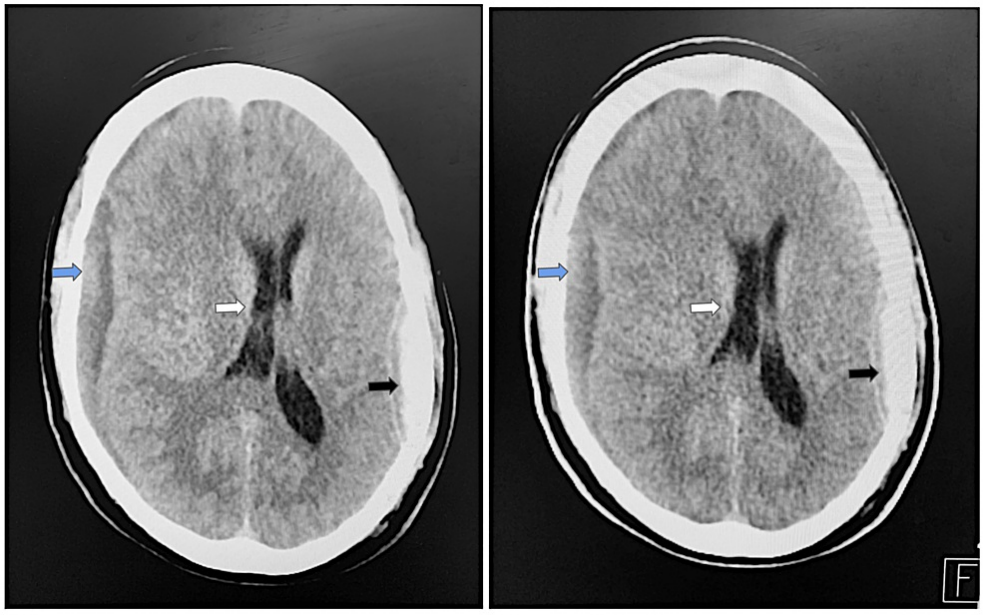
Day 1 of the second admission: second series of head CT scans after seizure. Radiology report revealed a stable subdural bleed (blue arrows) with right to left midline shift, stable epidural hematoma projecting over the left hemisphere (black arrows), and slightly increased local mass effect of the right frontal and temporal lobes with displacement of midline structures to the left with subfalcine herniation (white arrows).
